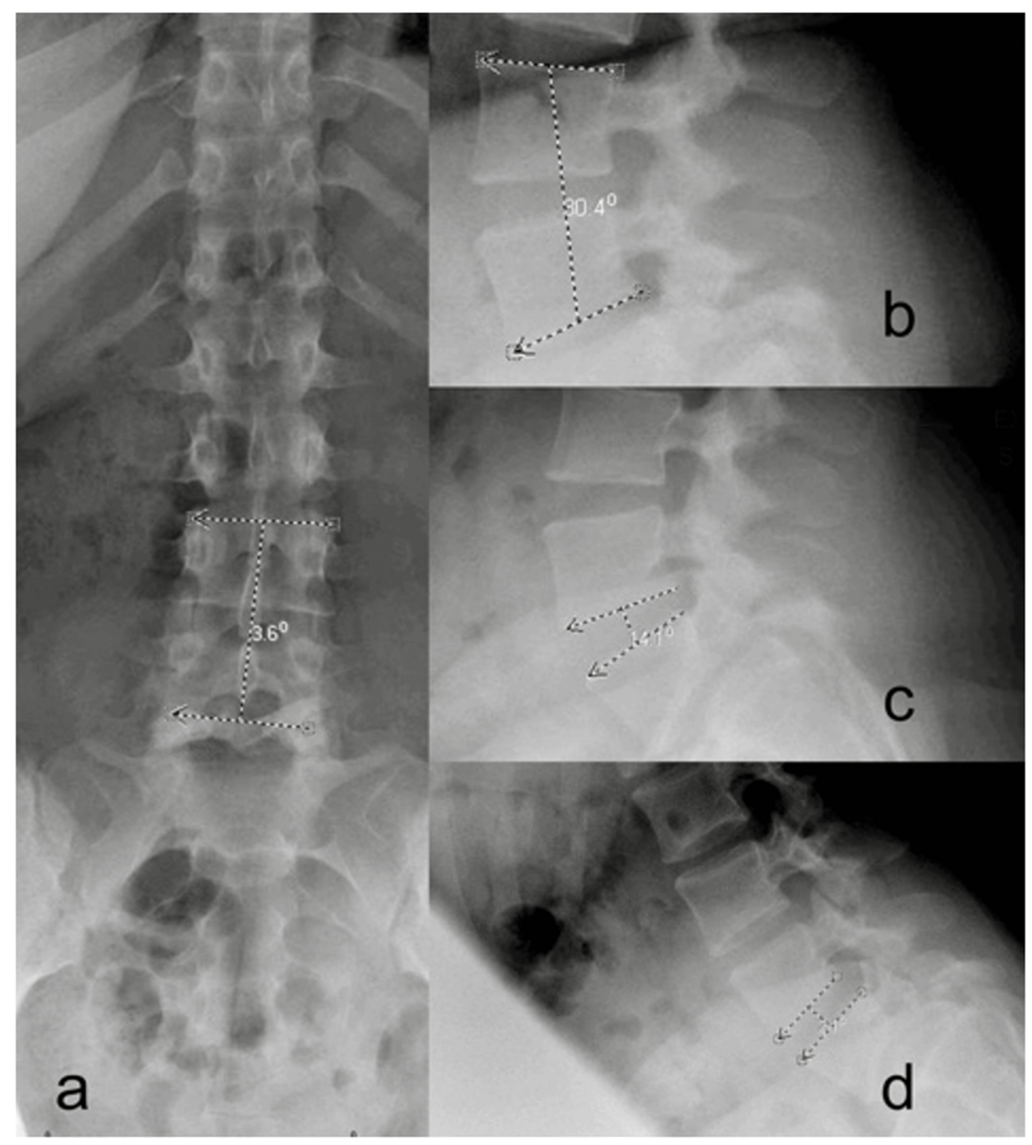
Pre-operative measurements on lateral standing X-ray. (a) Anterior-posterior view. (b-d) lateral view Source: Image: © 2024, Seattle Science Foundation. Used with permission from the institution solely for this manuscript
